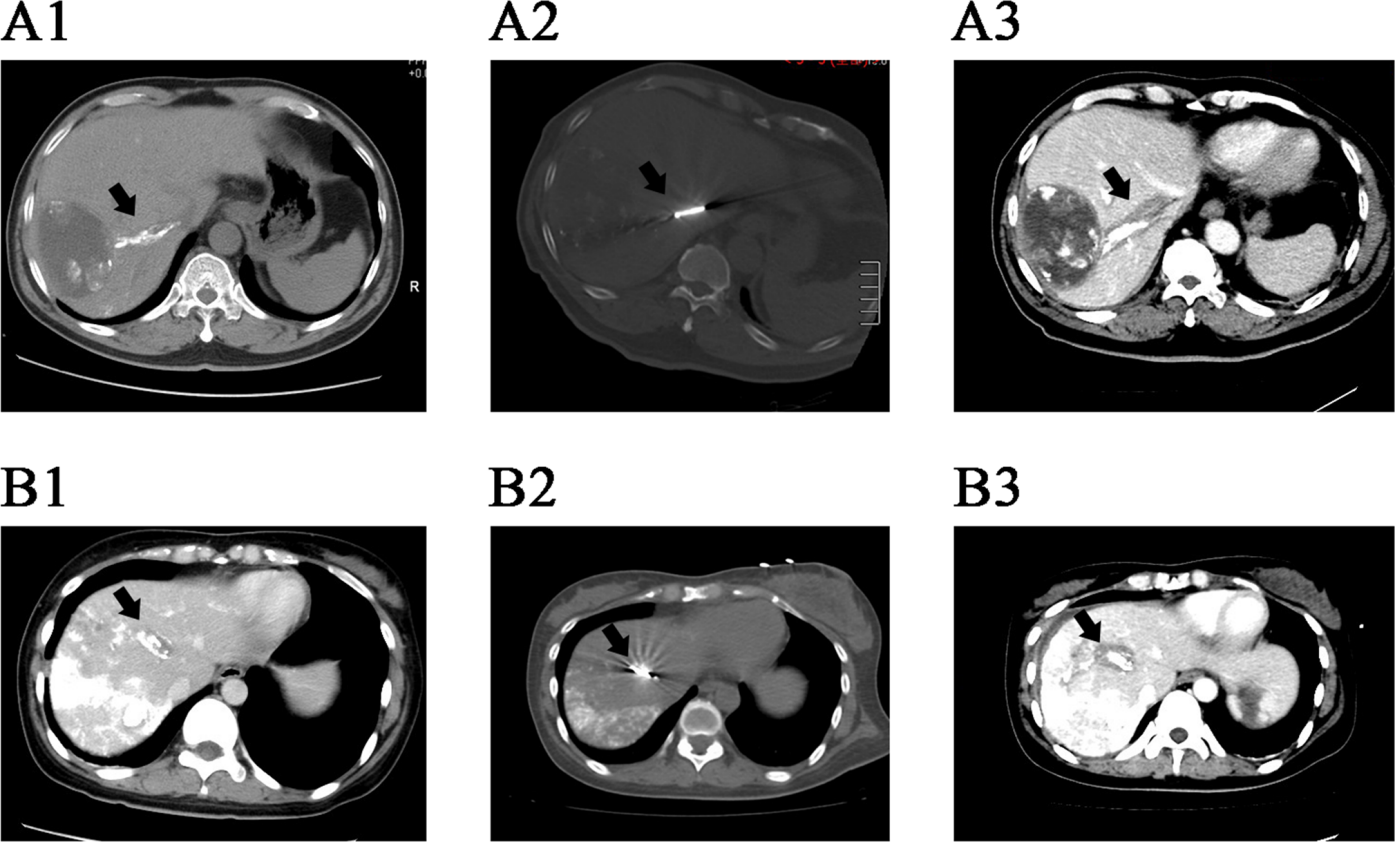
CT scanning of two patients with HVTT. (A1) CT scan of patient A after transcatheter arterial embolization showed tumor thrombus in the right hepatic vein. Lipiodol deposition in the thrombus feeding artery labeled the thrombus on CT imaging. (A2) Intra-operative CT scan of the ablation of thrombus. (A3) Instant post-operative contrast-enhanced CT of patient A showed complete necrosis of the thrombus: the thrombus was completely surrounded by the low density ablation zone. (B1) CT scan of patient B showed tumor thrombus and lipiodol deposition in the middle hepatic vein. (B2) Intra-operative CT scan of the ablation of thrombus. (B3) Instant post-operative contrast-enhanced CT of patient B showed that the thrombus was completely surrounded by the low density ablation zone. CT, computed tomography; HVTT, hepatic vein tumor thrombus.
